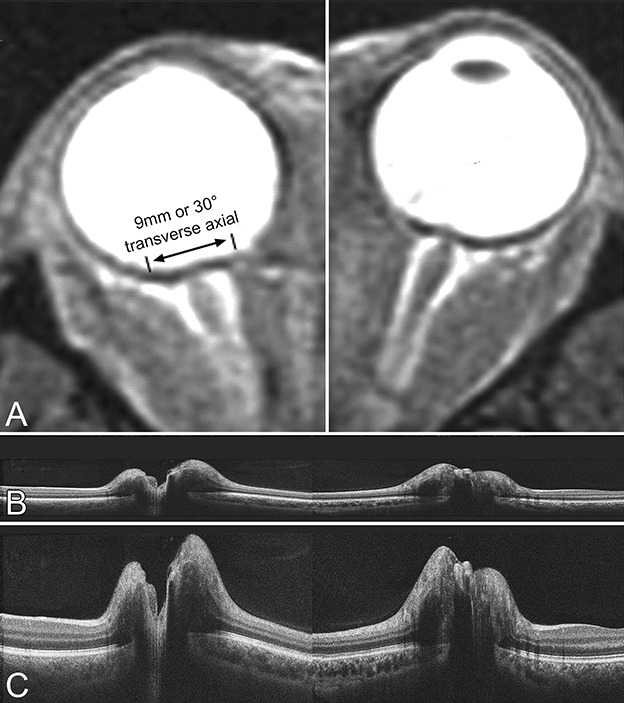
A. MRI showing distended optic nerve sheaths with flattening of the globe. Corresponding (B) unstretched transverse axial OCT and (C) 3× vertically scaled transverse axial OCT. OCT, optical coherence tomography.
